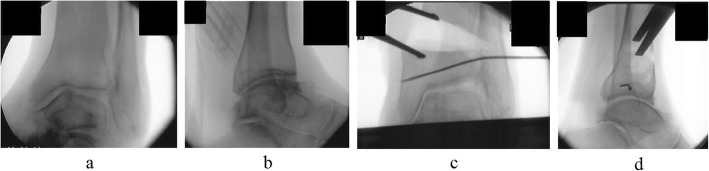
Osteotomy fluoroscopic images. a Varus tilt of the talus on anteroposterior fluoroscopic imaging before osteotomy. b Lateral fluoroscopic image before osteotomy. c Fluoroscopic image after osteotomy. The deformity has been corrected in the coronal plane. d Fluoroscopic image taken after osteotomy. Correction is performed until the radiographic signs of subluxation disappear on lateral fluoroscopy imaging
